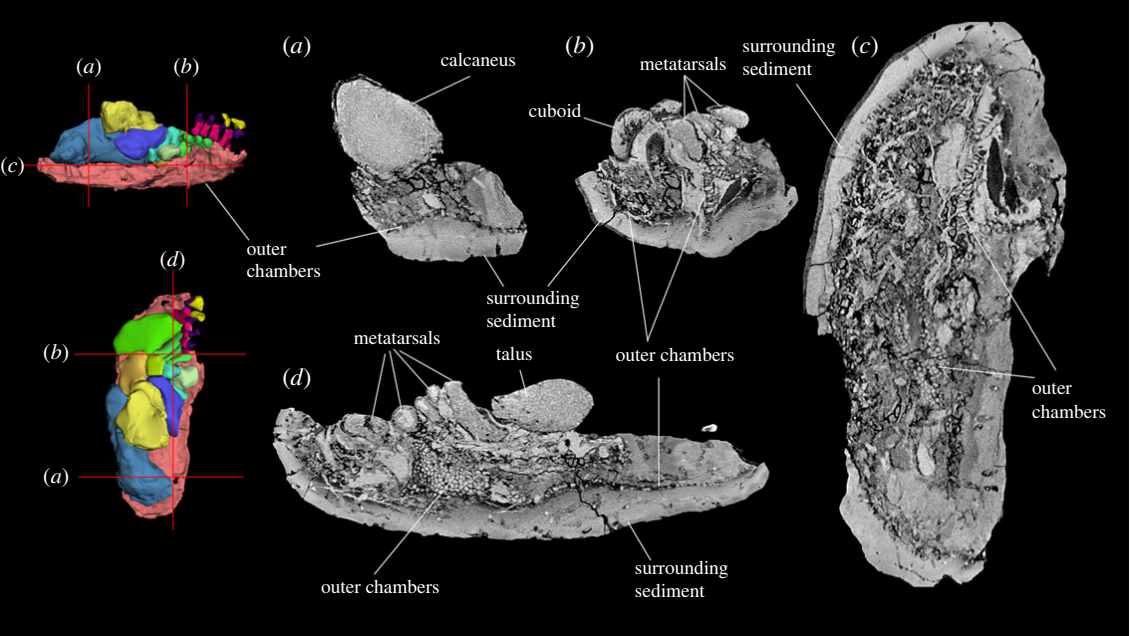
Cross-sectional images from the CT scan of the left pes of *Ambulator keanei* (SAMA P54742) (not to scale).
